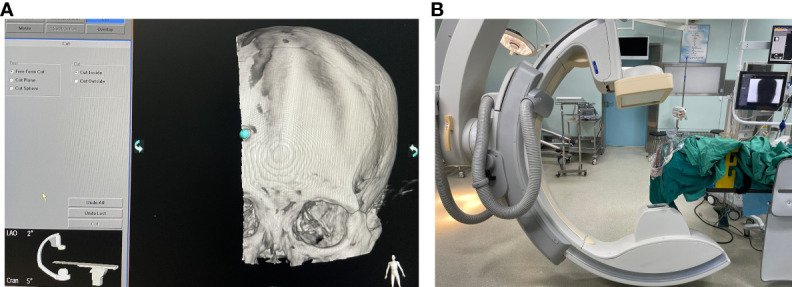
(A) Setting the puncture angle. (B) Xper CT rotation positioning.
